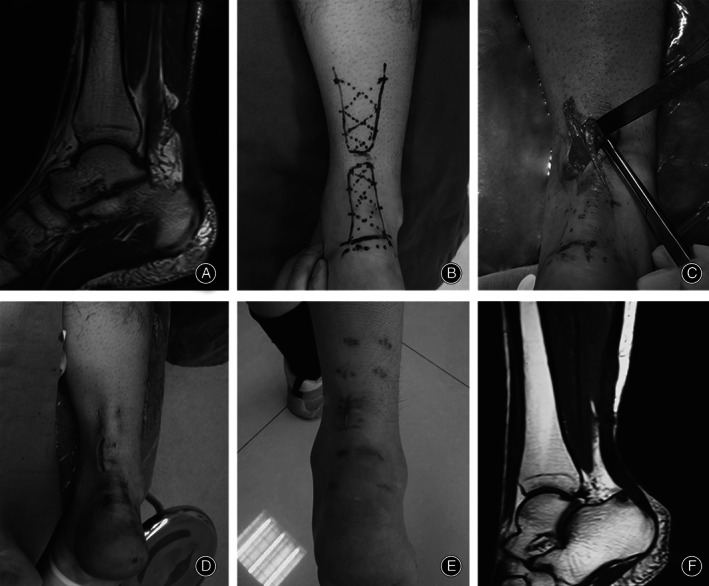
A 31‐year‐old male patient with ruptured Achilles tendon due to playing badminton. (A) Preoperative right lower limb MRI showed rupture of Achilles tendon; (B) before the operation, plan the suture route; (C) exposure of the broken end of the Achilles tendon during the operation; (D) before the incision is closed, a small incision can be seen; (E, F) postoperative MRI showed that the Achilles tendon had been repaired. During the follow‐up 6 months after operation, the incision was very small and the man returned to normal life.
